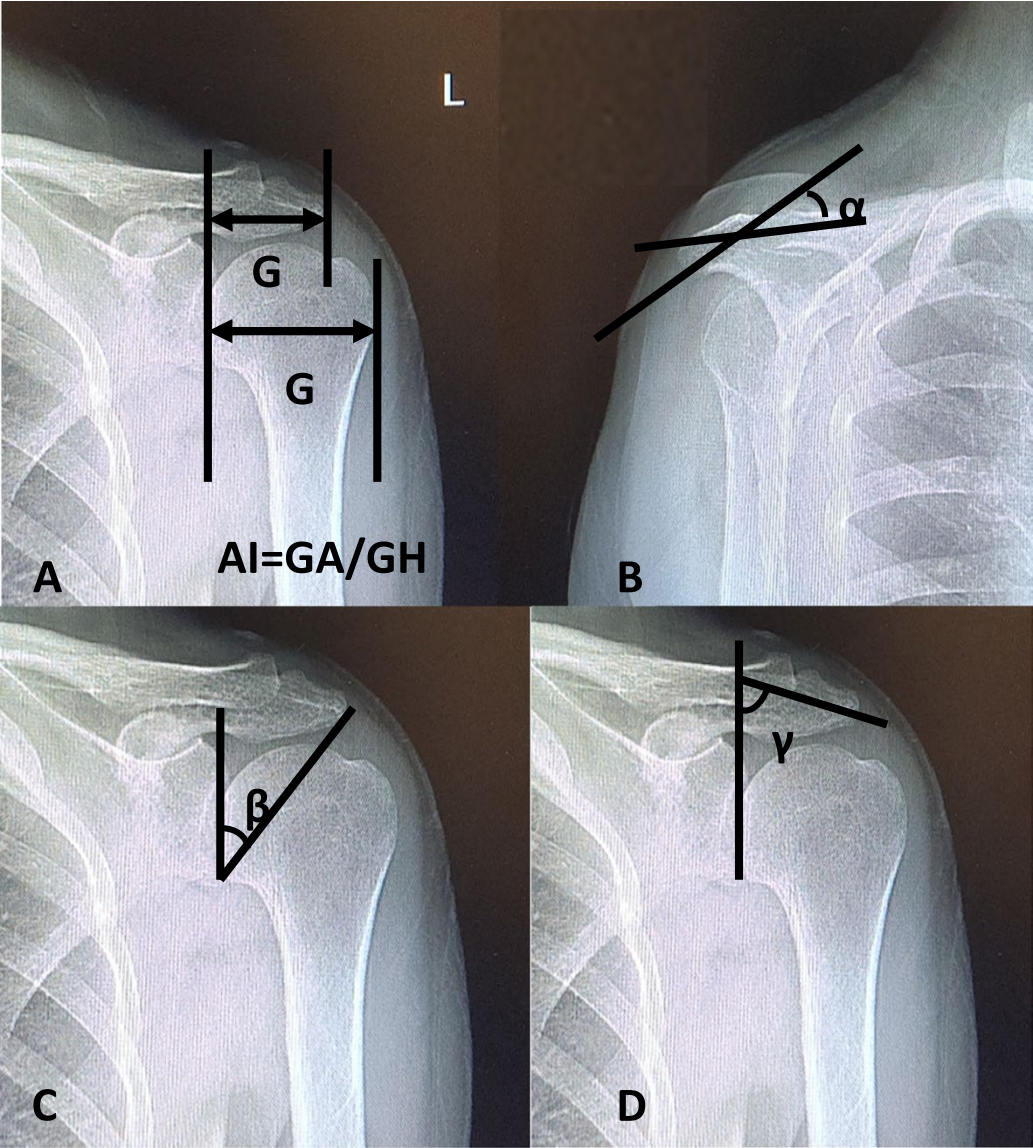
Radiographic parameters of shoulder joint parameters. A acromion index, B acromion slope, C critical shoulder angle, D lateral acromial angle
